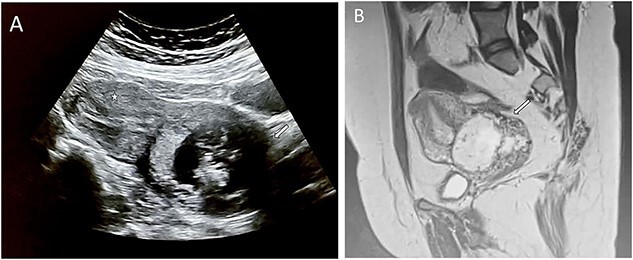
(A) Endovaginal ultrasound showing an evolving intracervical pregnancy of 11 amenorrhea weeks (arrow). (B) Magnetic resonance imaging confirming the endocervical location of the pregnancy with thinning of the posterior cervical stroma (arrow).
